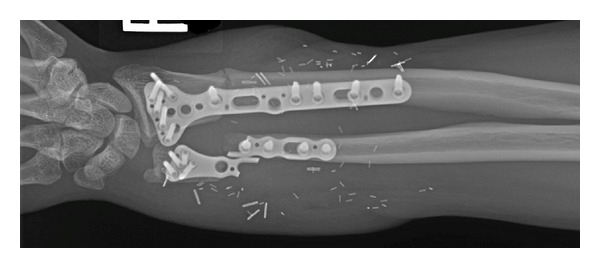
Radiograph showing development of nonunion and failed hardware.
